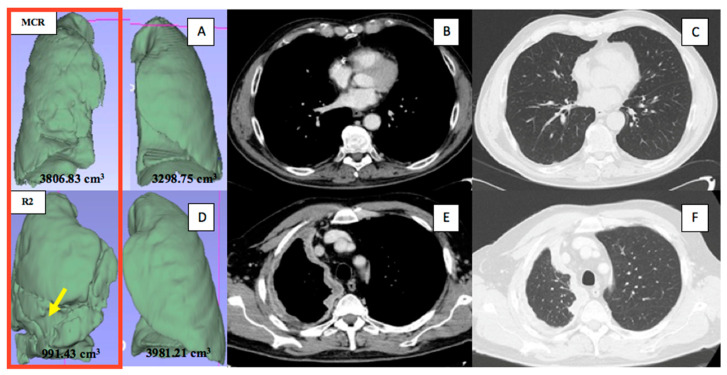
Representative radiological measurements. Upper panels, a patient with a right-sided MPM affected by the lowest disease burden, who underwent macroscopic complete resection: the aerated ipsilateral and contralateral lung volumes (A) and the pleural thickness (too small to measure) in the axial CT images on the portal venous phase (B) and the lung window (C), at the medium level. Lower panels, a patient with a right-sided MPM affected by the highest disease burden (the arrow indicated the furrows on the three-dimensional lung reconstruction caused by the pleural disease), who underwent R2 resection: the aerated ipsilateral and contralateral lung volumes (D) and the pleural thickness in the axial CT images on the portal venous phase (E) and the lung window (F), at the upper level.
